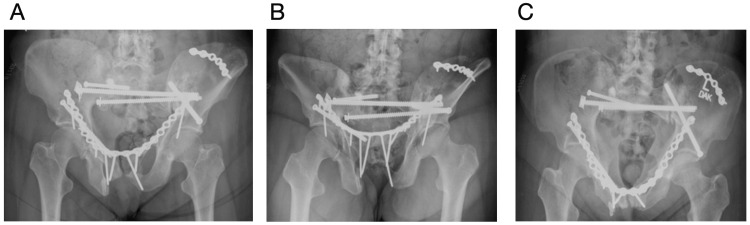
Revision surgery post op radiographs Images A (AP pelvis), B (pelvic outlet), and C (pelvic inlet) demonstrate removal of the previous broken S1 transsacral screw with exchange to a larger cannulated screw in the same S1 transsacral corridor, as well as an additional screw in the S1 iliosacral corridor. An antegrade anterior column cannulated screw was also placed to provide additional support to anterior column.
